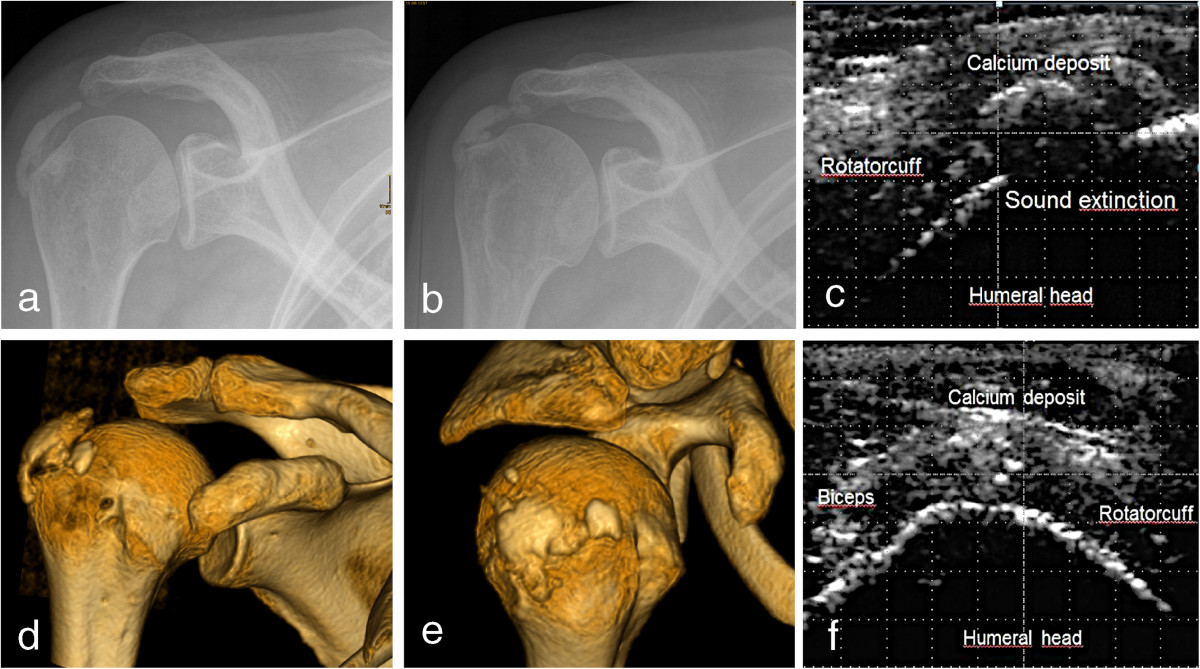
Imaging of calcium deposit in calcifying tendinitis with conventional X-rays, ultrasound and computed tomography. Conventional X-rays (a+b), ultrasound (c+f) and computed tomography (d-e) of the same patient suffering a calcifying tendinitis of the rotator cuff. The Ultrasound image of the patient presented with full sound extinction (c). Underneath a CD without sound extinction is shown (f).
